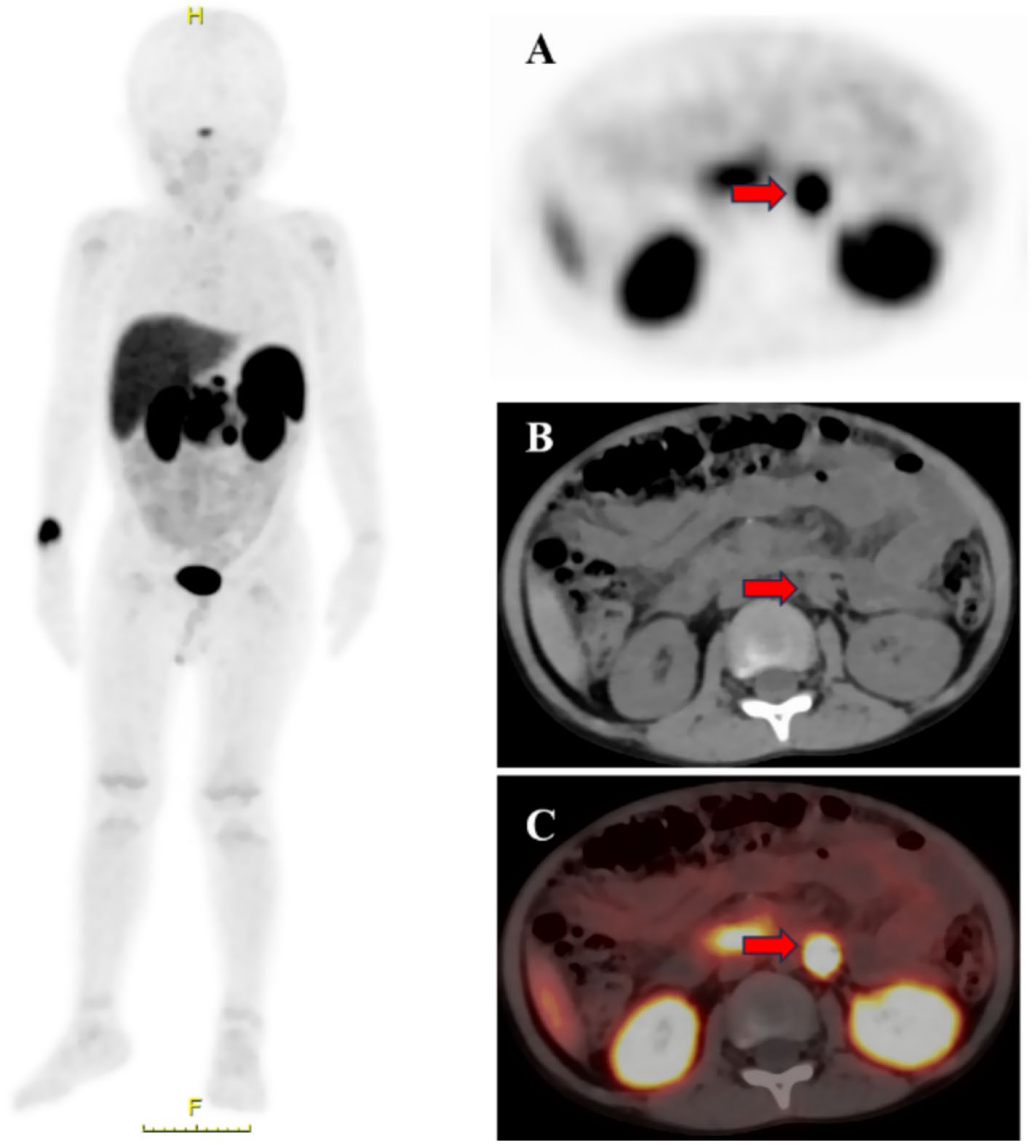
The primary site was located in the retroperitoneum in a child (male, 4 years old), PET showed [(A), red arrow] a rounded hyperintense focus in the left adrenal region; CT on the same machine showed [(B), red arrow] a rounded nodular focus in the left adrenal region with a diameter of approximately 0.9 cm; PET/CT showed [(C), red arrow] a rounded hyperintense focus in the left adrenal region with an SUVmax of 17.9. The final reopening postoperative pathology the diagnosis of ganglion cell neuroma was confirmed.
